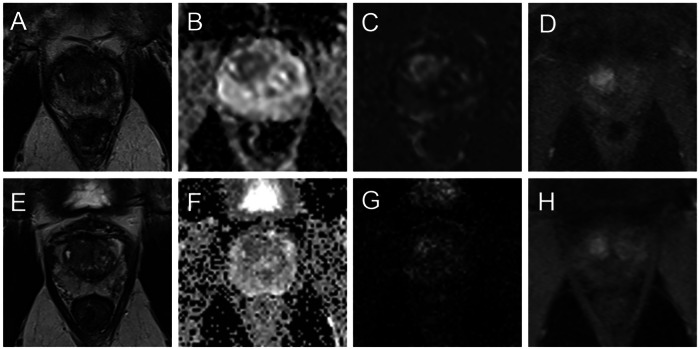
72 age, Status post MRI/TRUS-fusion biopsy (05/2021; 0/12 systematic cores; 2/6 targeted cores, TZa apex right side/5a; max. infiltration 90%; Gleason score 3 + 3 = 6, ISUP GG1). Rising PSA-level (PSA recently 15 ng/ml; previously 04/2021 PSA 12.7 ng/ml). Prostate volume 41 ml, constant 41 ml (04/2021). PSA density 0.37 ng/ml/ml, previously 0.30 ng/ml/ml (04/2021). Comparison of recent MRI A–D and first MRI (E–H). Progression of the anterior lesion. PI-RADS (v2.1): 5. PRECISE-Score (v2): 4 MR Staging: cT2c Follow-up biopsy: ISUP GG 2 (80% core infiltration) Radical prostatectomy: pT2c pN0 (0/13) L0 V0 Pn0 R0 ISUP GG 2.
